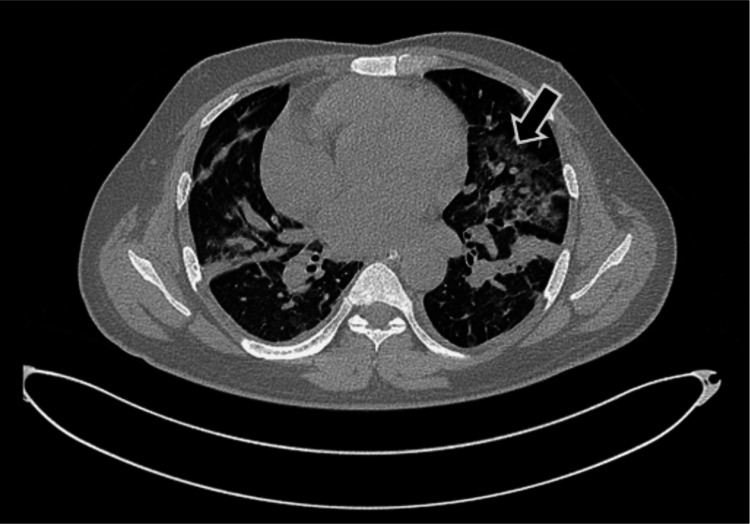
High-resolution computed tomography (HRCT) of the chest (axial view) showing bilateral patchy areas of ground-glass opacities (arrow). These findings are suggestive of non-cardiogenic pulmonary edema, a rare complication of hemolytic uremic syndrome (HUS), although some changes may also reflect pre-existing chronic lung pathology.
